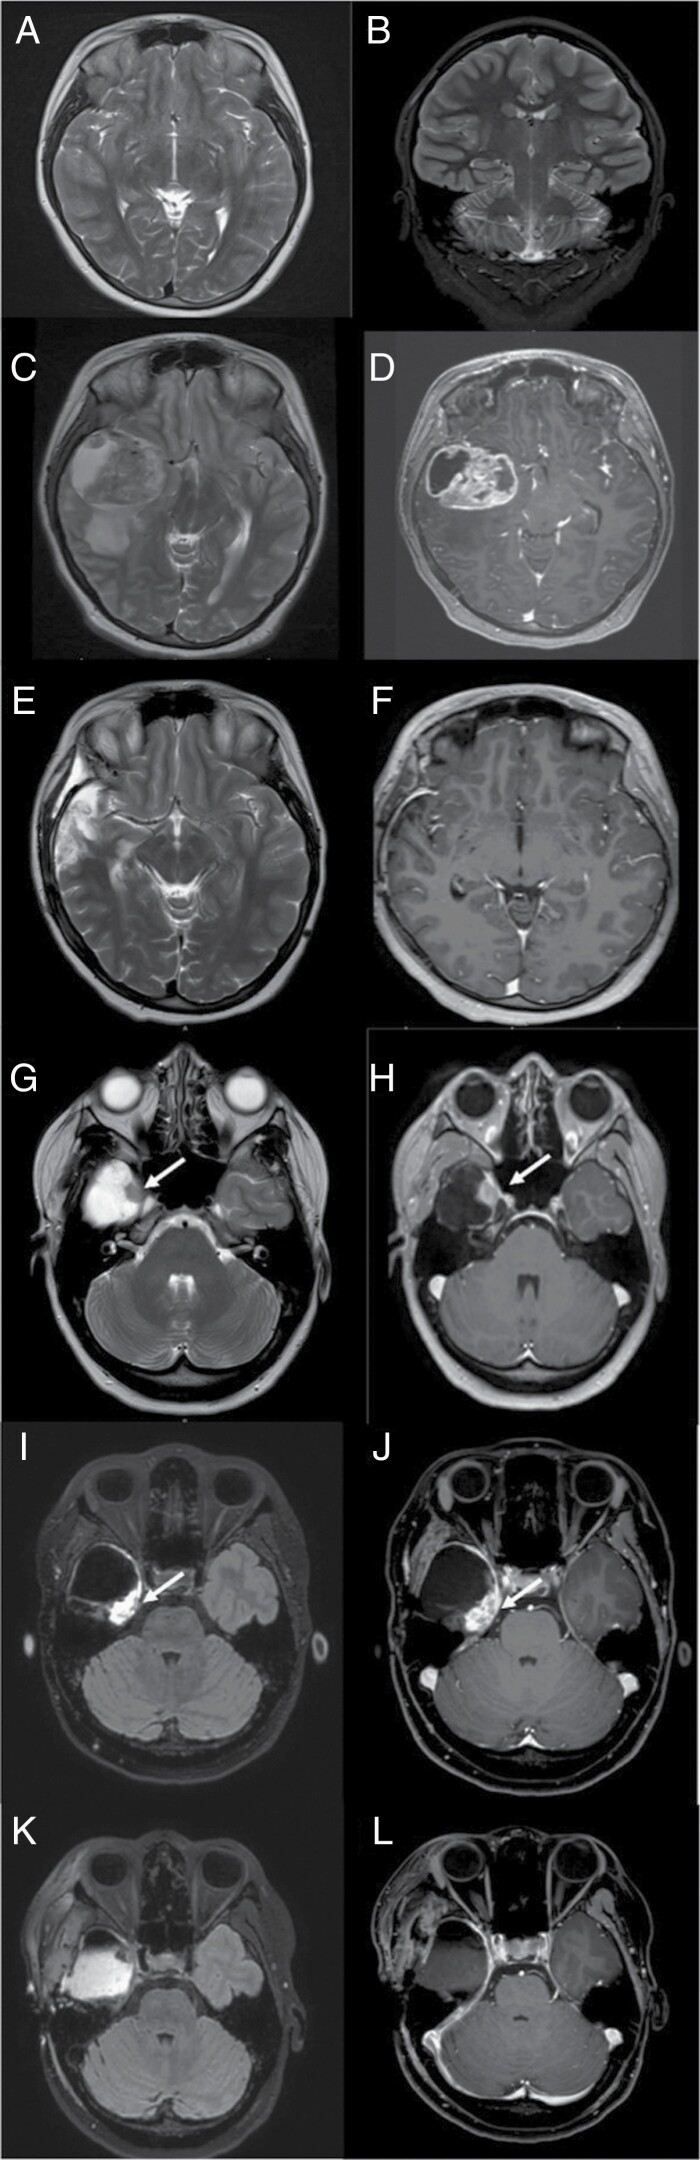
Brain magnetic resonance imaging (MRI) at the time of epilepsy diagnosis. No lesion was identified (axial T2 and coronal T2-A and B). Brain MRI 20 months later with a large right temporal lobe lesion with mass effect and vasogenic edema (axial T2 and axial T1 with gadolinium-C and D). Post-surgery brain MRI showing near total resection (axial T2 and axial T1 with gadolinium-E and F). Brain MRI demonstrating local recurrence after 32 months of treatment (arrows) (axial T2 and axial T1 with gadolinium-G and H). Brain MRI demonstrating the second local recurrence after 47 months of treatment (arrows) (axial T2 Flair and axial T1 with gadolinium-I and J). Post-surgery brain MRI showing a gross total resection (axial T2 Flair and axial T1 with gadolinium-K and L).
